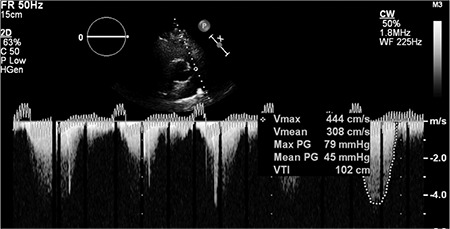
Continuous wave Doppler of the pulmonary valve.
